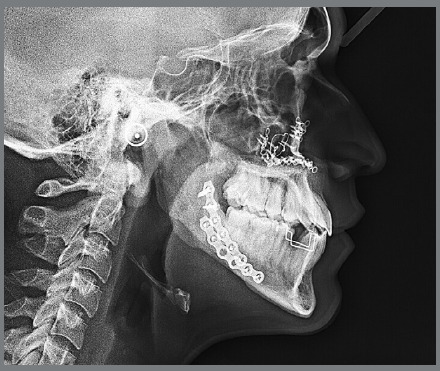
Final lateral radiograph.
